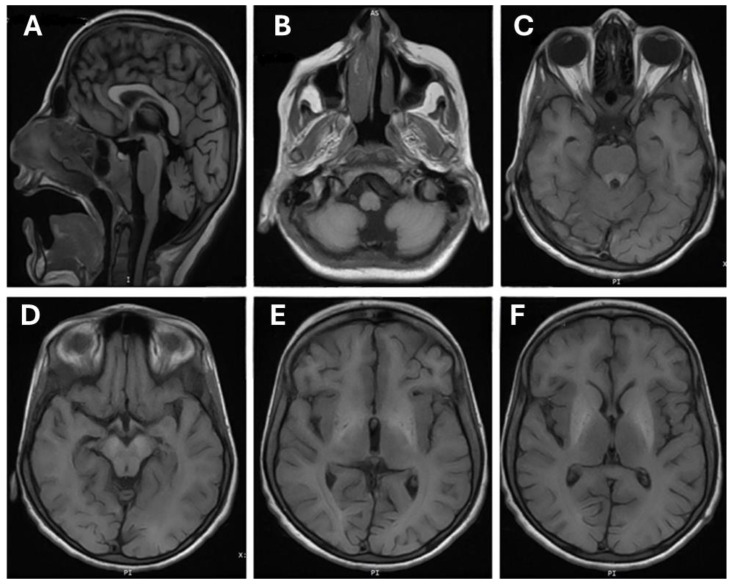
Brain magnetic resonance imaging (MRI) of patient (III-3). (A) Cerebellar atrophy flair saggital sequence flair; (B) hypoplasia of vermis axial T1; (C) increased signal from the basal ganglia down to the brain stem mainly midbrain (cerebri) and pones (ventral). Ventrical pons around 4th ventricle; (D) midbrain (cerebri bilateral); (E) frontal bilateral white matter hypersignal; (F) continuation of hypersignal to basal ganglia signs of hypomyelination.
